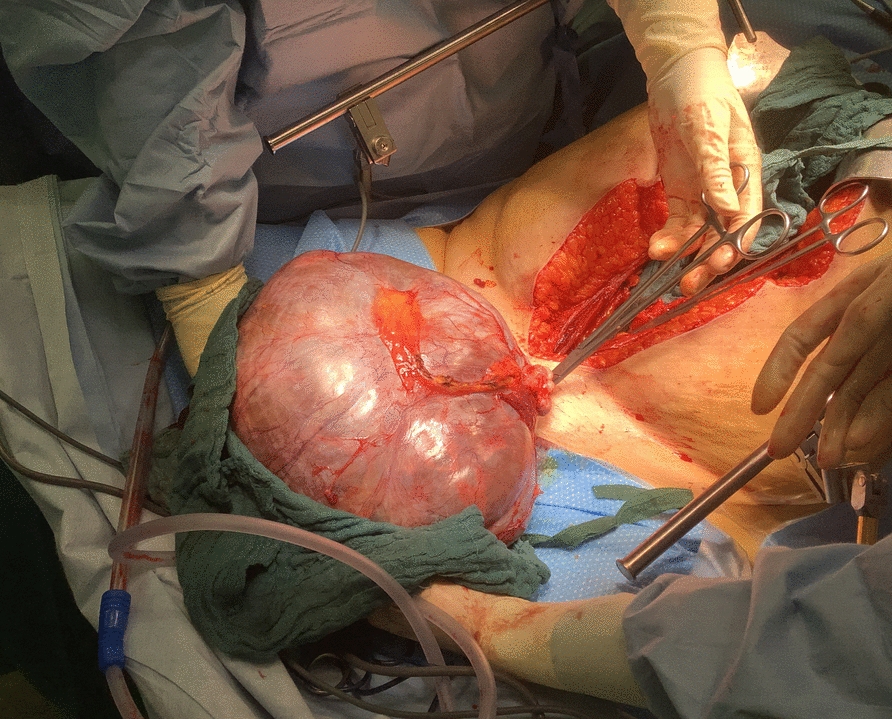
Tumor of the left ovary which was detected by positron emission tomography–computed tomography and had shown elevated glucose utilization at the margins. Pathological diagnosis: borderline tumor of the ovary with transition into endometrioid ovarian cancer
